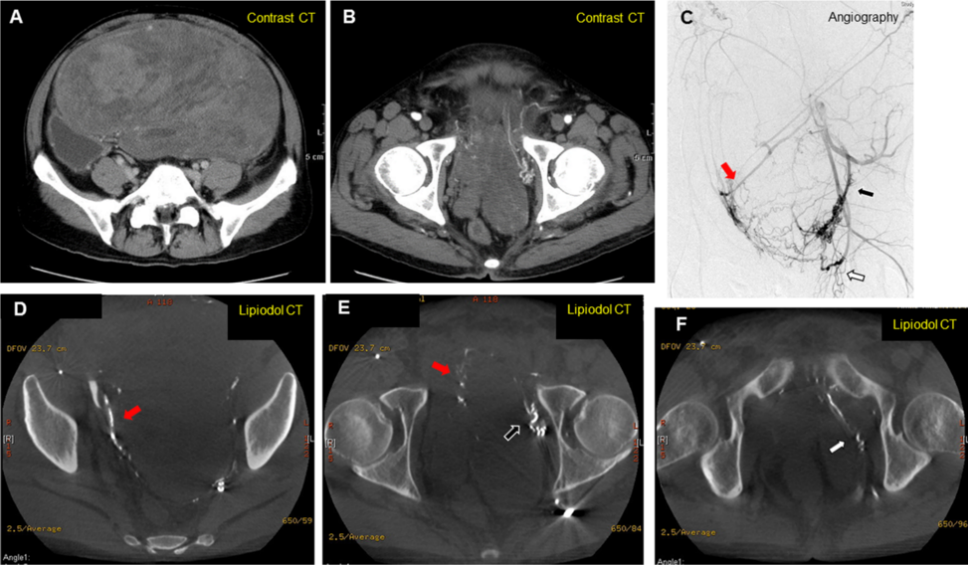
Preoperative computed tomography (CT), angiography before embolization, and plain CT after embolization with lipiodol and *N*-butyl-2-cyanoacrylate. (A), (B) Preoperative CT showed a giant pelvic mass lesion, measuring 30 × 25 × 19 cm. (C) Angiography of the left iliac artery revealed that the mass was mainly supplied by the left obturator artery (black arrow), left internal pudendal artery (white arrow), and left superior vesical artery (red arrow). (D), (E), (F) CT after embolization showed that lipiodol and *N*-butyl-2-cyanoacrylate were well distributed to the left obturator artery (black arrow), left internal pudendal artery (white arrow), and left superior vesical artery (red arrow), demonstrating successful embolization of the target vessels.
